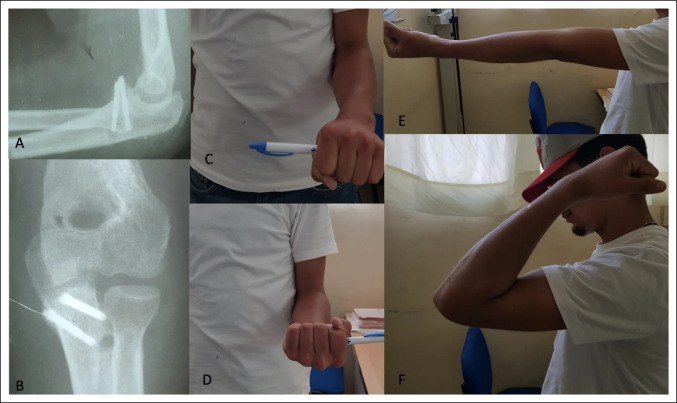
Follow-up at 18 months. A and B, Postsurgical AP and lateral radiographic view of our patient. C–F, Range of motion of our patient at the final follow-up.
